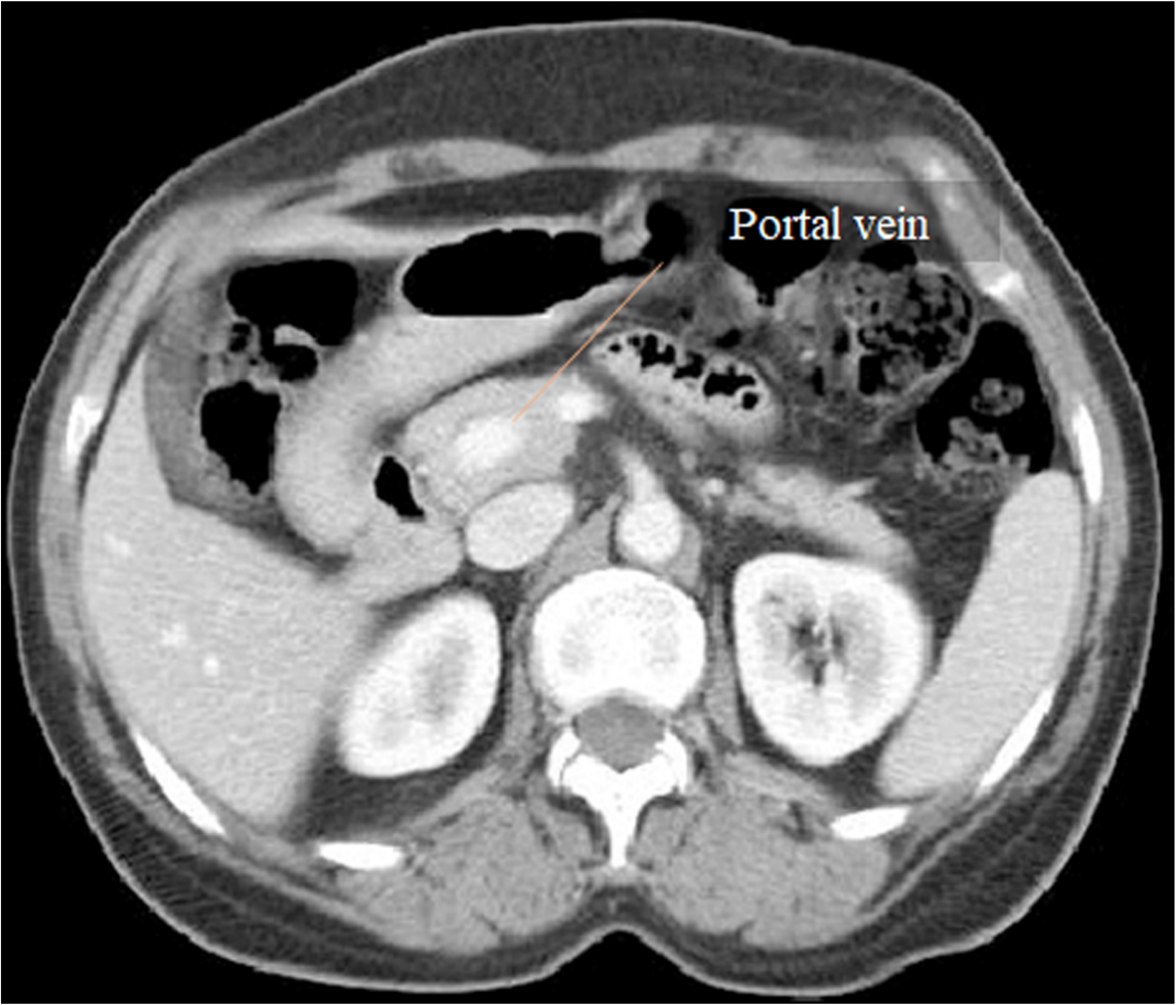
Circumportal pancreas. Portal venous phase CT axial image shows pancreatic parenchyma surrounding PV like an annulus. In this case, it was a suprasplenic type
